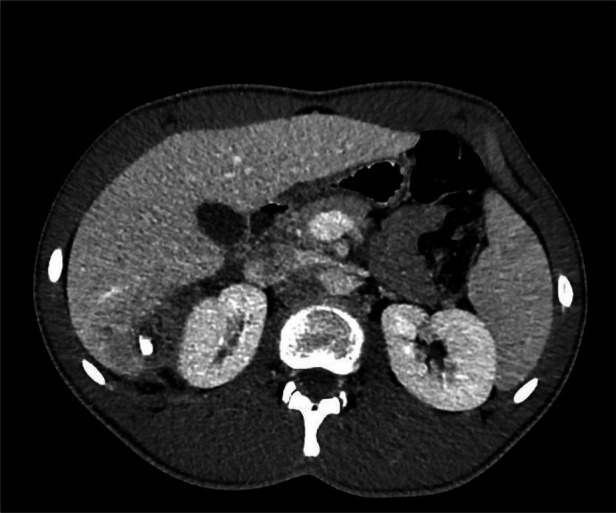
CT scan: Abscess in S6/S7 containing a hyperdense calcified element.
